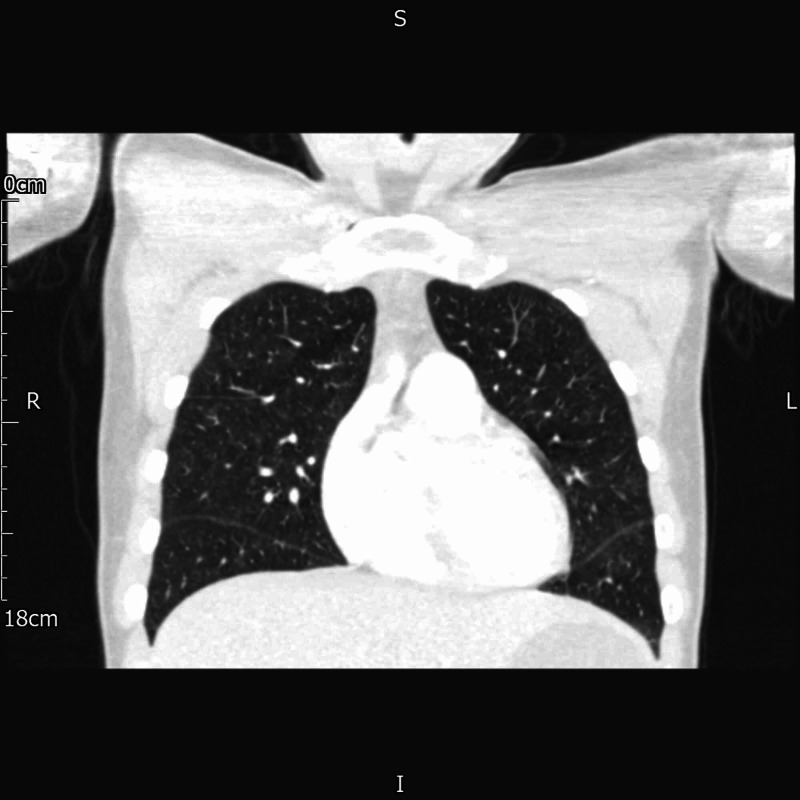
Coronal images of chest CT showing bilateral ground glass opacification.
